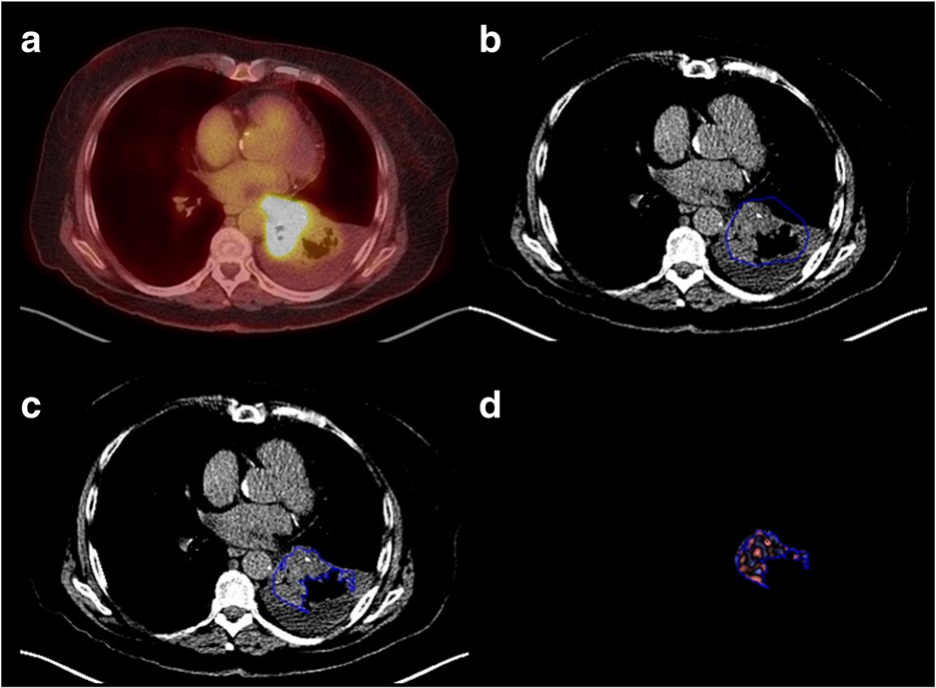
Left lower lobe NSCLC showing cavitation and adjacent consolidation. The fused FDG-PET/CT image (a) and narrow CT windows (b) can assist identification of the tumour margins. Using automatic segmentation, the initial manually constructed ROI (b) includes adjacent lung and the area of cavitation but excludes the adjacent mediastinal structures and pulmonary consolidation. The final ROI defined by the automated segmentation procedure (c) exlcudes the adjacent lung and area of cavitation. The final filtered tumour image is shown in (d)
